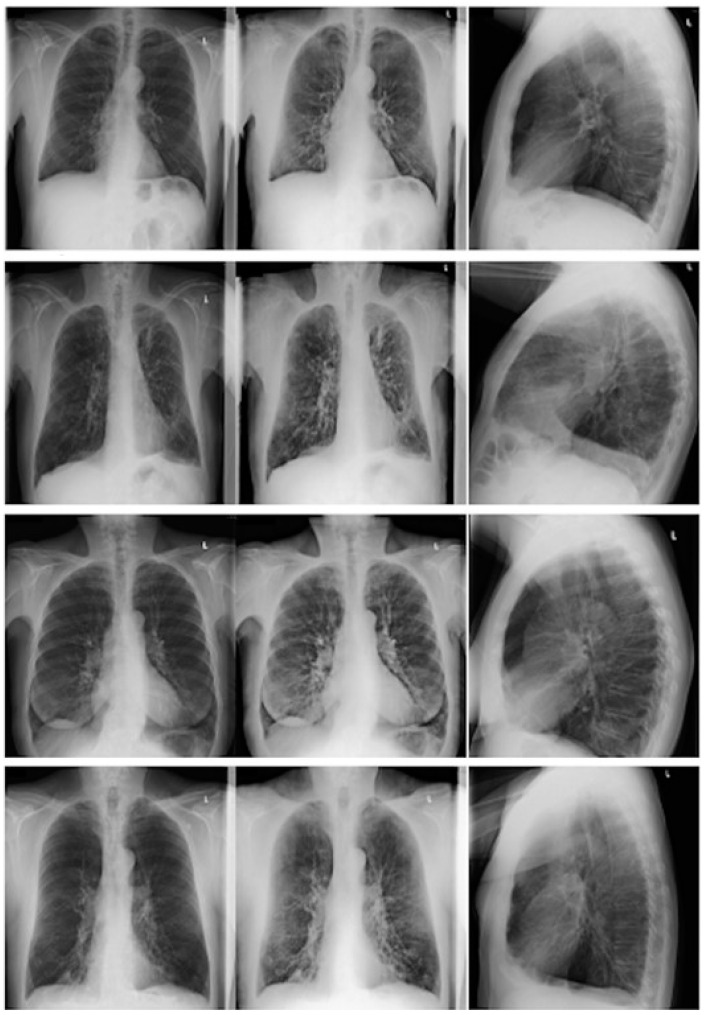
Four patients with varying degrees of emphysema in the right upper lobe.First row: no emphysema; second row: mild emphysema; third row: moderate emphysema; and fourth row: severe emphysema. Left column: conventional postero anterior (p.a.) x-ray; middle column: dual-energy p.a. x-ray; and right column: conventional lateral x-ray.
